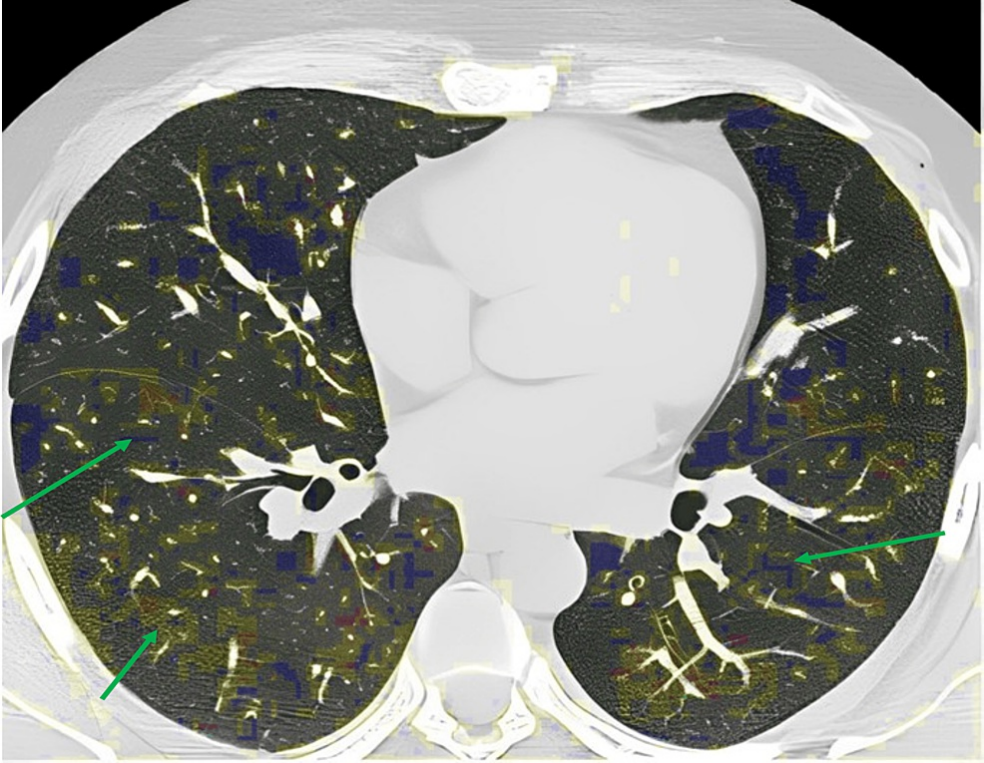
Axial chest CT scan after chemical treatment showed complete radiological clearance with the disappearance of all lung lesions (the arrows indicate the reduction or disappearance of lesion areas after treatment).
